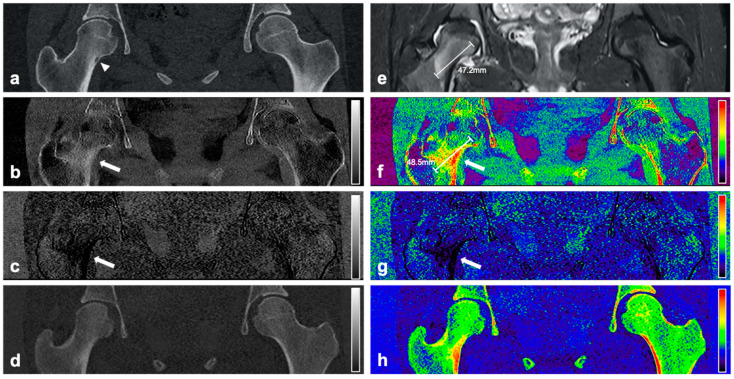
CT and MR images of an 18-year-old female patient with intracortical OO in the right femoral neck. Coronal reformation of the conventional CT examination (a) reveals the nidus in the right femoral neck with surrounding sclerosis (arrowhead). Material-specific volume fraction maps for red marrow/water (b), yellow marrow/fat (c), and hydroxyapatite (d), and respective color-coded overlays (f–h) reveal an increase in water-specific volume fraction in the red-marrow map (b,f; arrow) and a decrease in fat-specific volume fraction in the yellow-marrow map (c,g; arrow) in the right femoral neck, compared to the respective area on the other side. The corresponding coronal STIR MR image confirms the presence of a half-moon shaped edema-equivalent signal alteration in the right femoral neck around (“half-moon sign” [13]) (e). Calipers indicate measurements of the extent of BME.
